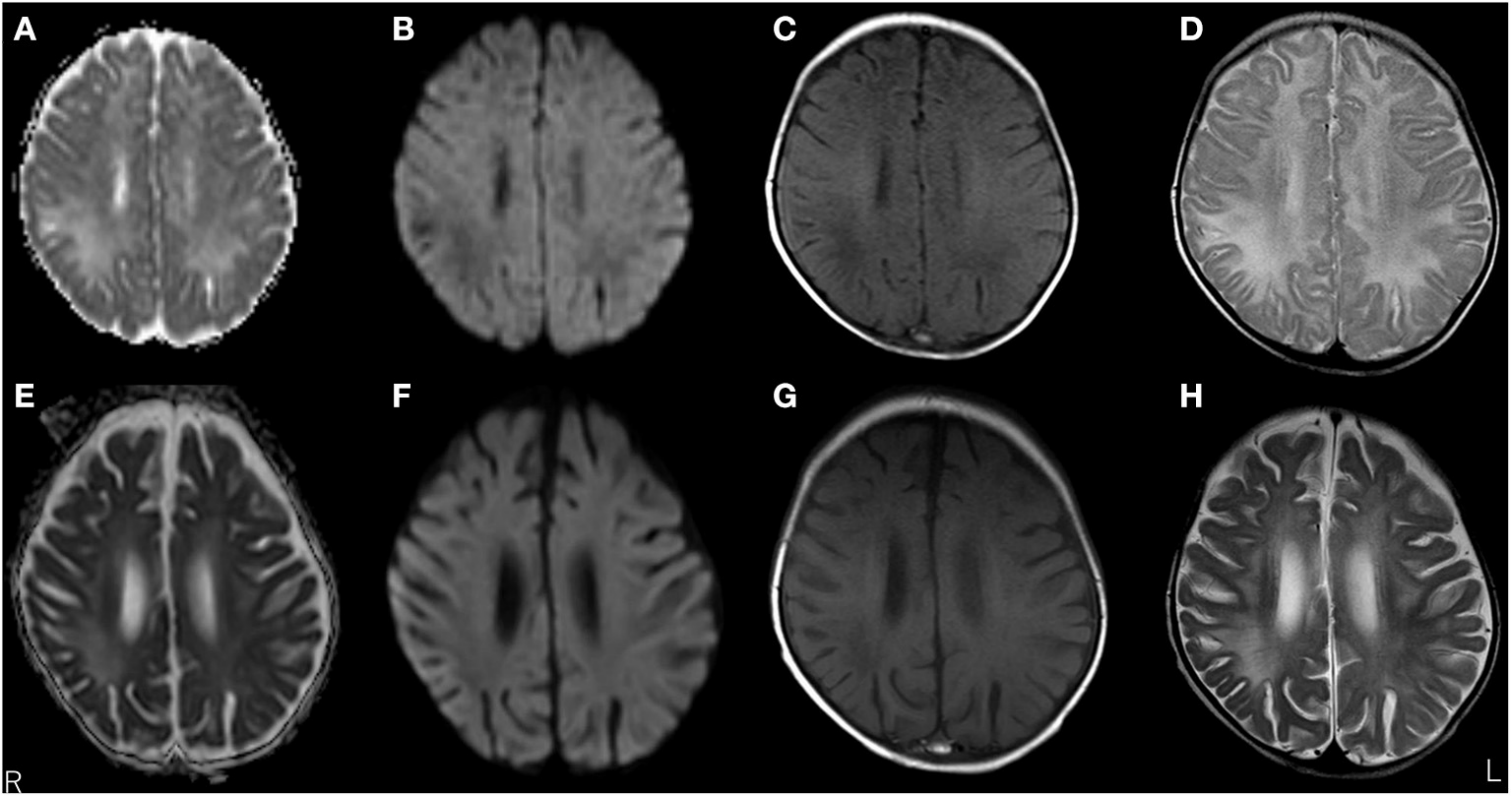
Brain magnetic resonance imaging (MRI) over time. MRI at 3 months old showed white matter lesions with high intensity on apparent diffusion coefficient maps (A) and T2 images (D), and low intensity on diffusion-weighted (DW) images (B) and T1-weighted images (C) within the right frontal and parietal lobes and both occipital lobes, suggesting demyelination. MRI at 5 months old revealed a significant decrease in white matter volume with slight improvements in abnormal lesions within the right frontal, left parietal, and left occipital lobes. No remarkable changes were observed in lesions within the right occipital lobe with high intensity on apparent diffusion coefficient maps (E) and T2-weighted images (H), and low intensity on DW images (F) and T1-weighted images (G).
